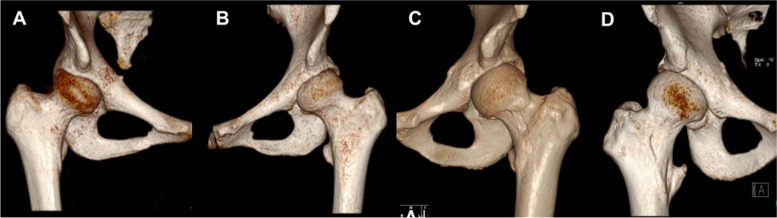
Type I (A), Type IIA (B), Type IIB (C) and Type III (D) AIISs Imaged by 3-D CT
